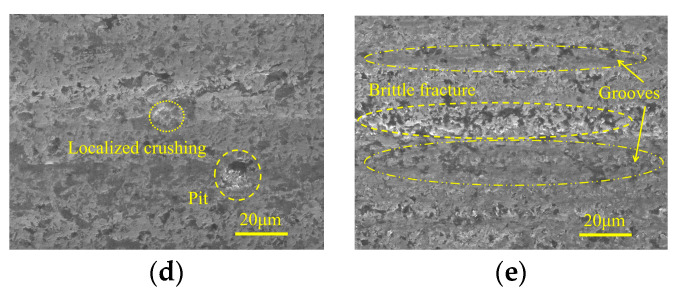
Micro-morphologies under different ultrasonic amplitudes in semi-finishing grinding: (a) $T_{A}$: 0 μm $W_{A}$: 4 μm; (b) $T_{A}$: 4 μm $W_{A}$: 4 μm; (c) $T_{A}$: 8 μm $W_{A}$: 4 μm; (d) $W_{A}$: 0 μm $T_{A}$: 4 μm; and (e) $W_{A}$: 8 μm $T_{A}$: 4 μm.
